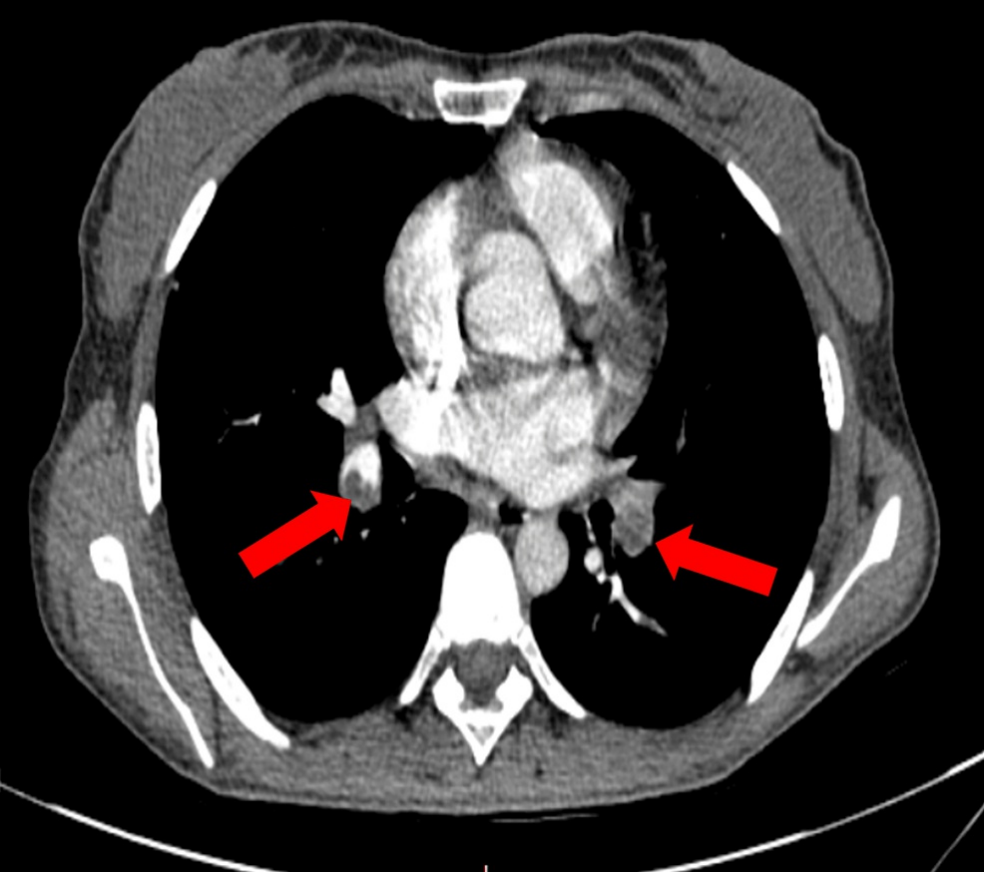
Thorax CT showing bilateral pulmonary artery thrombus with filling defect (red arrows)
